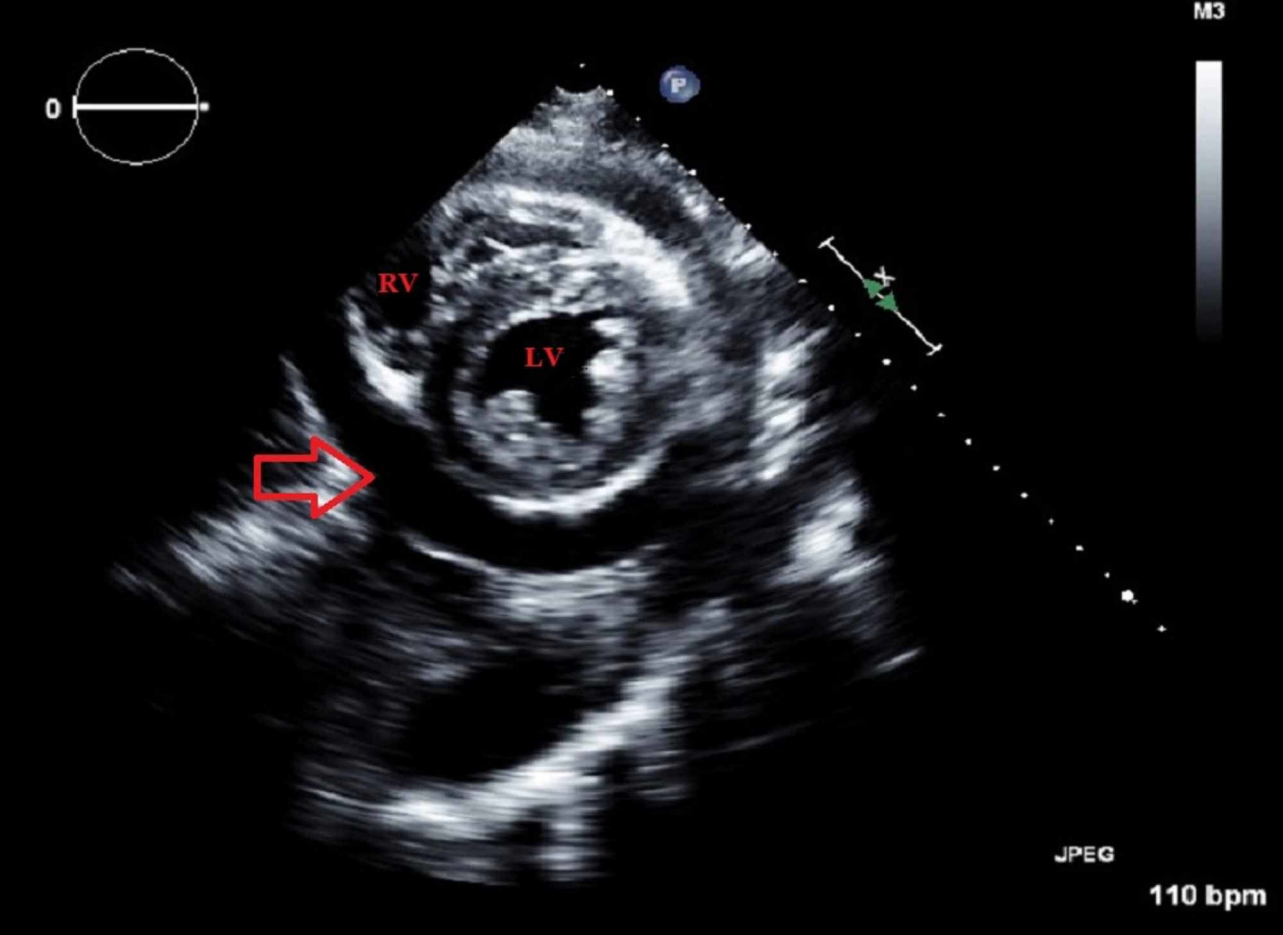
Short-axis TEE view, showing the left ventricle, right ventricle and a moderate-to-large pericardial effusion (arrow). TEE: Transthoracic echocardiogram
